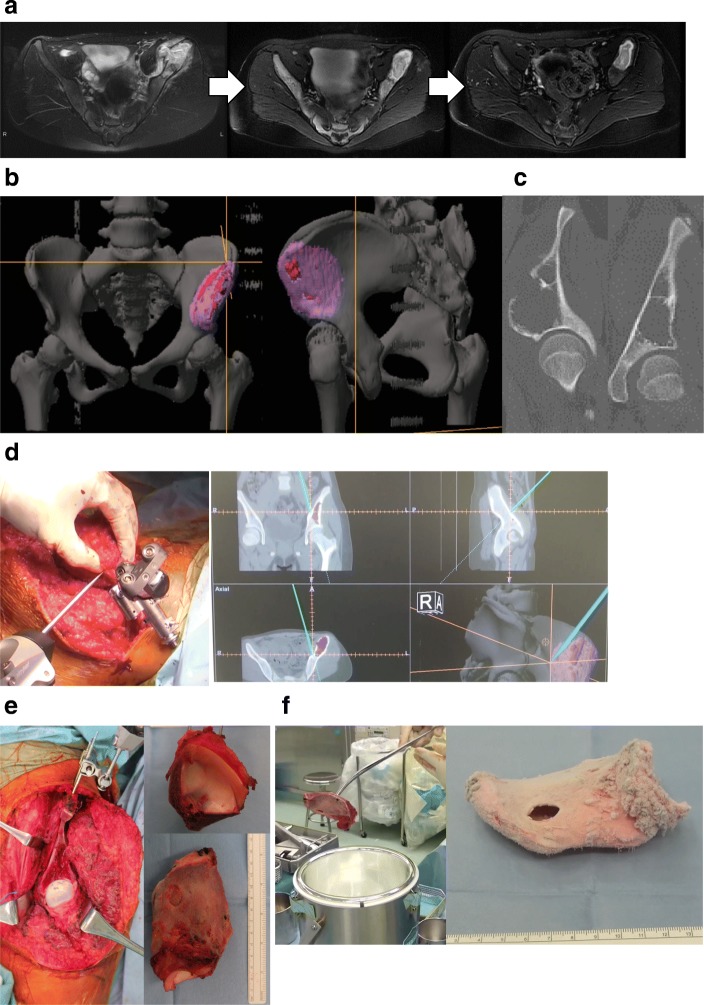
Preoperative assessments in case 1: A 12-year-old girl with Ewing sarcoma of the pelvis (PI-II). a Preoperative magnetic resonance imaging. b) Preoperative plan involving the use of a computer navigation system. c) Preoperative computed tomography. d Checking the margin using a computer navigation system. e After osteotomy. f Freezing in liquid nitrogen
